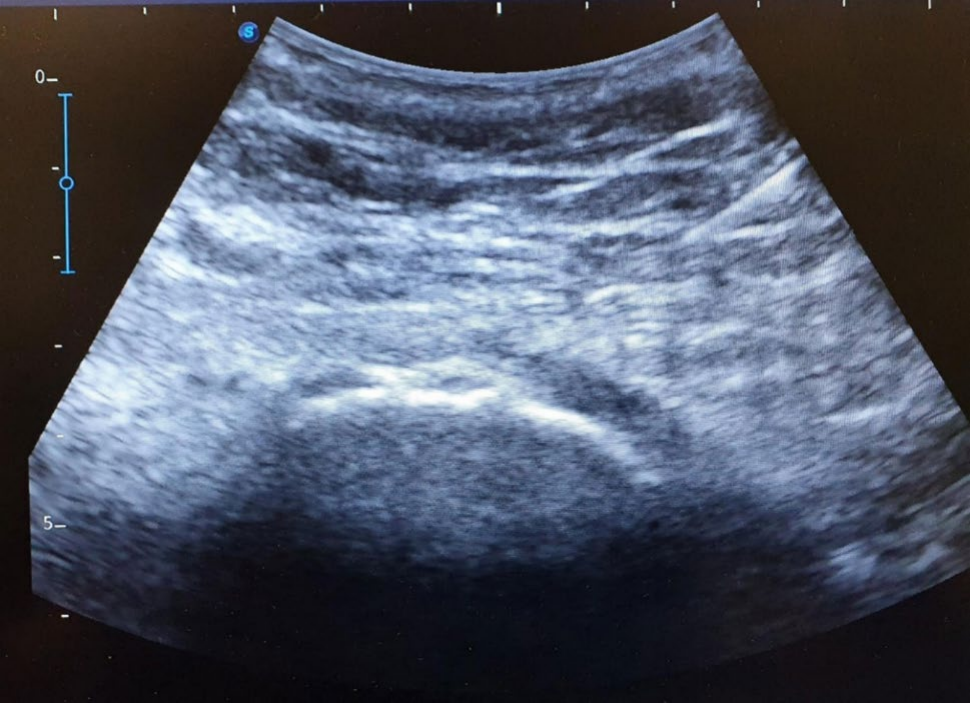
Transverse ultrasound image
